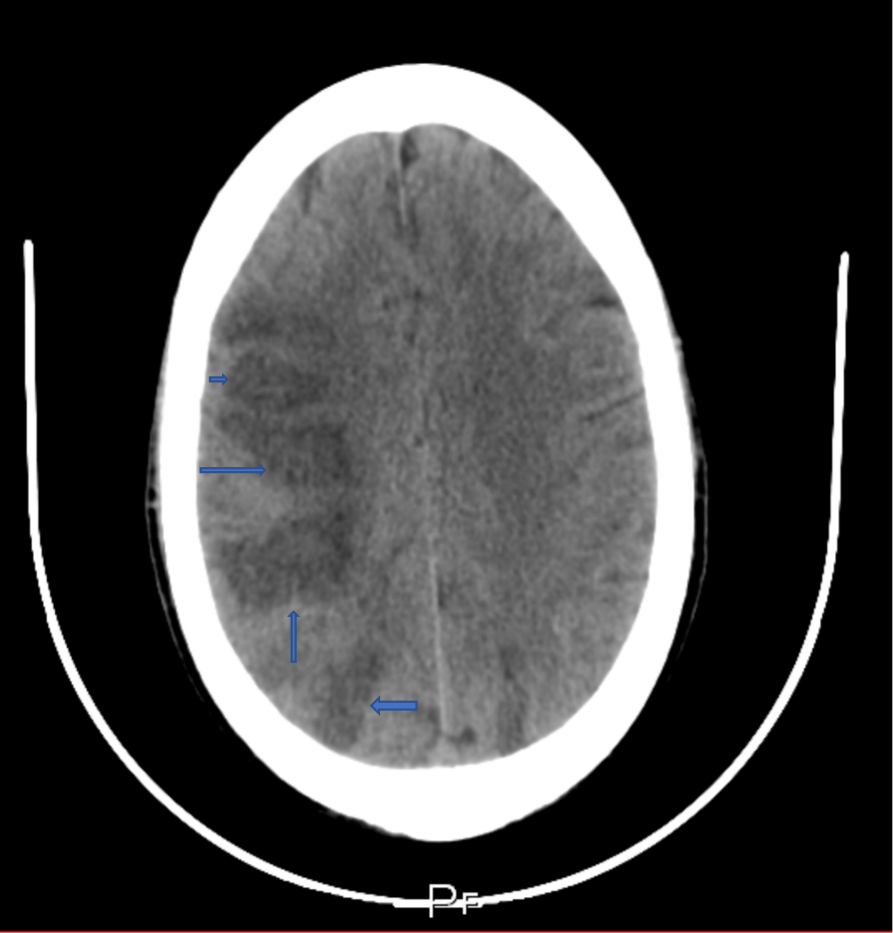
Repeat non-contrast CT scan of the brain with newly developed multiple right parieto-temporal hypodensities
